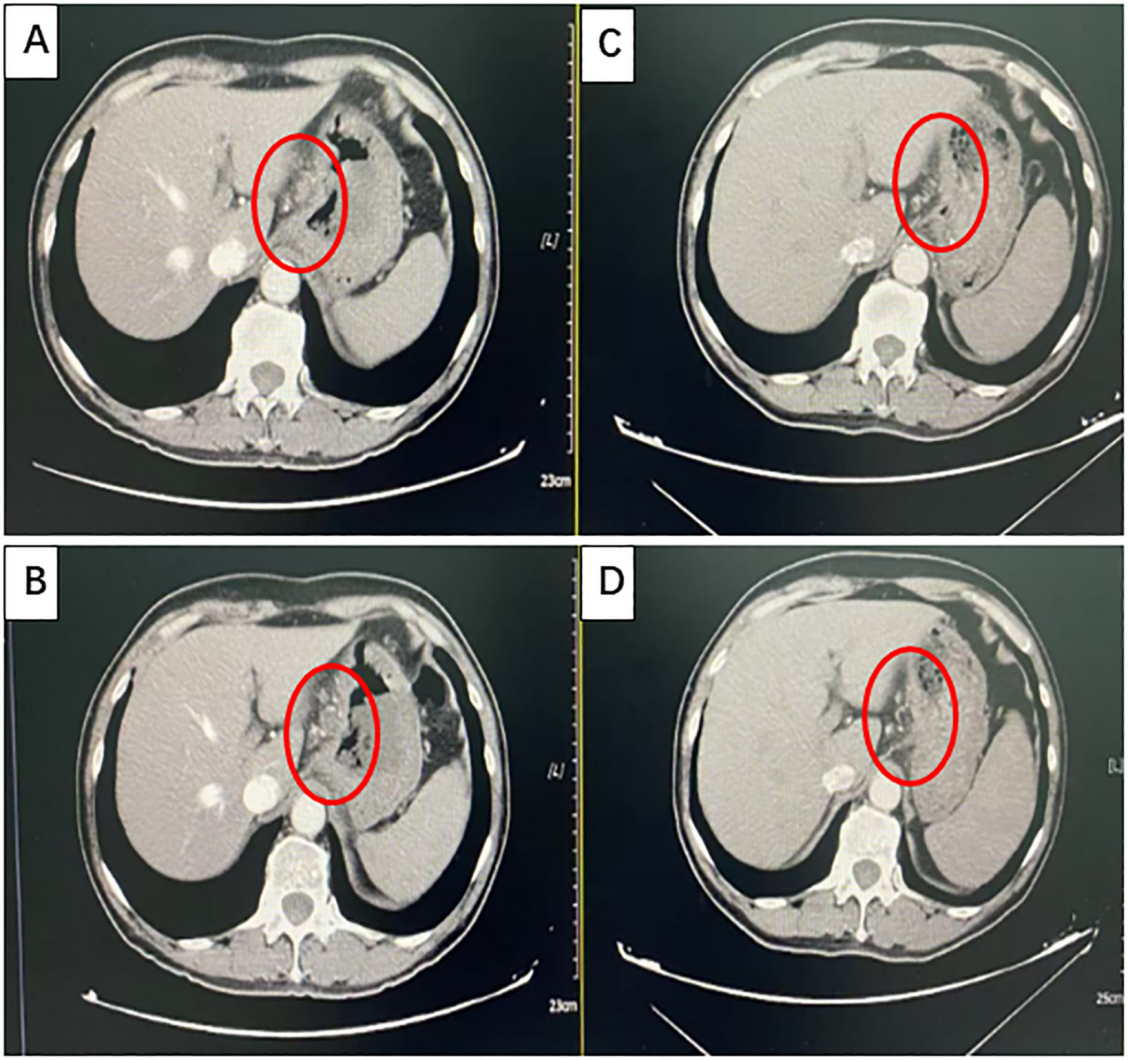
Partial response (PR) was confirmed using computed tomography (CT). (A, B) Images taken at baseline before treatment initiation. (C, D) Partial response (PR) was assessed using CT after three cycles of treatment (primary foci and abdominal lymph node metastatic lesions were markedly decreased).
